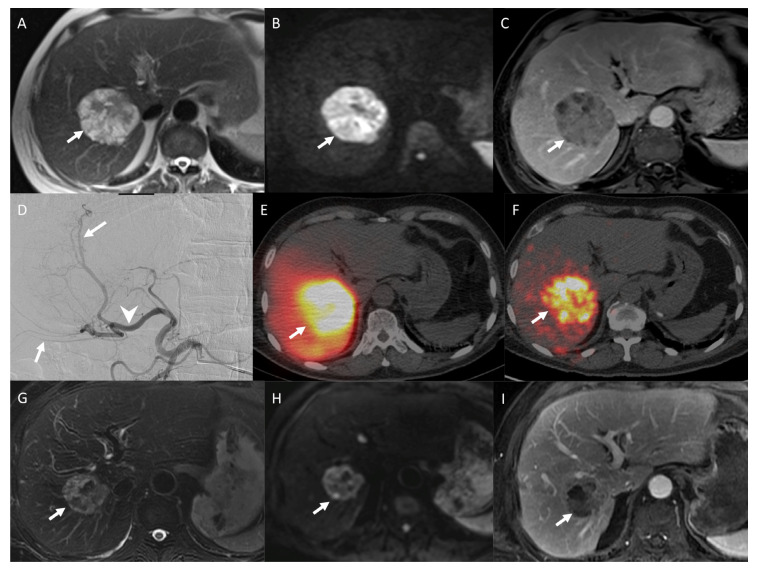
An 80-year-old woman midgut neuroendocrine tumor grade I (Ki67 = 1%), treated 10 years ago with surgery. She had an exacerbation of symptoms resistant to octreotide. MRI examination obtained before selective internal radiation therapy (SIRT) shows a single, hyperintense, and heterogeneous metastasis of the right liver (arrow) on T2-weighted image (A), marked diffusion restriction on high b-value diffusion-weighted image (arrow) (B), and heterogeneous enhancement on T1-weighted MR image obtained after intravenous administration of a gadolinium chelate during the arterial phase (arrow) (C). Arteriogram of common hepatic artery shows two feeding arteries (arrows) originating from the right branch of the hepatic artery (arrowhead). The injection point was defined 15 mm upstream to arterial bifurcation (D). Pre-treatment scintigraphy after injection of macroaggregated albumin labeled with 99m-Technetium shows an important uptake by metastasis (arrow), a low uptake by adjacent healthy liver, and no non-target embolization (E). Post-SIRT scintigraphy confirmed these results, with uptake by metastasis (arrow) (F). One year after SIRT, MRI shows a decrease in lesion size (2.5 versus 6 cm) (arrow), on T2-weighted image (G), a decrease in diffusion restriction (arrow) (H), and no more hyperenhancement on T1-weighted MR image after injection of a gadolinium chelate during the arterial phase (arrow) (I). Patient has no recurrent disease during the first two years after SIRT. Then, she had a complementary treatment by lutetium Lu 177 DOATATE (Lutathera^®^, Novartis, Basel, Switzerland). She is still alive to date.
